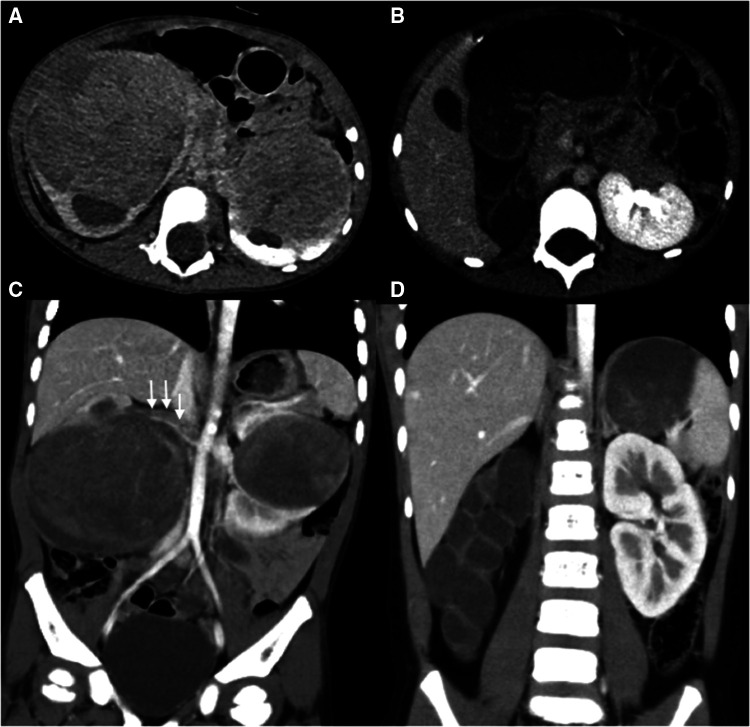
Abdominal CT scans of case 8. (A,C) Preoperative CT images reveal bilateral kidney lesions invading the renal sinus with bilateral hydronephrosis, a filling defect is noted within the right renal vein and inferior vena cava (white arrow). (B,D) Postoperative CT images reveal the absence of right kidney and Normal left morphology.
